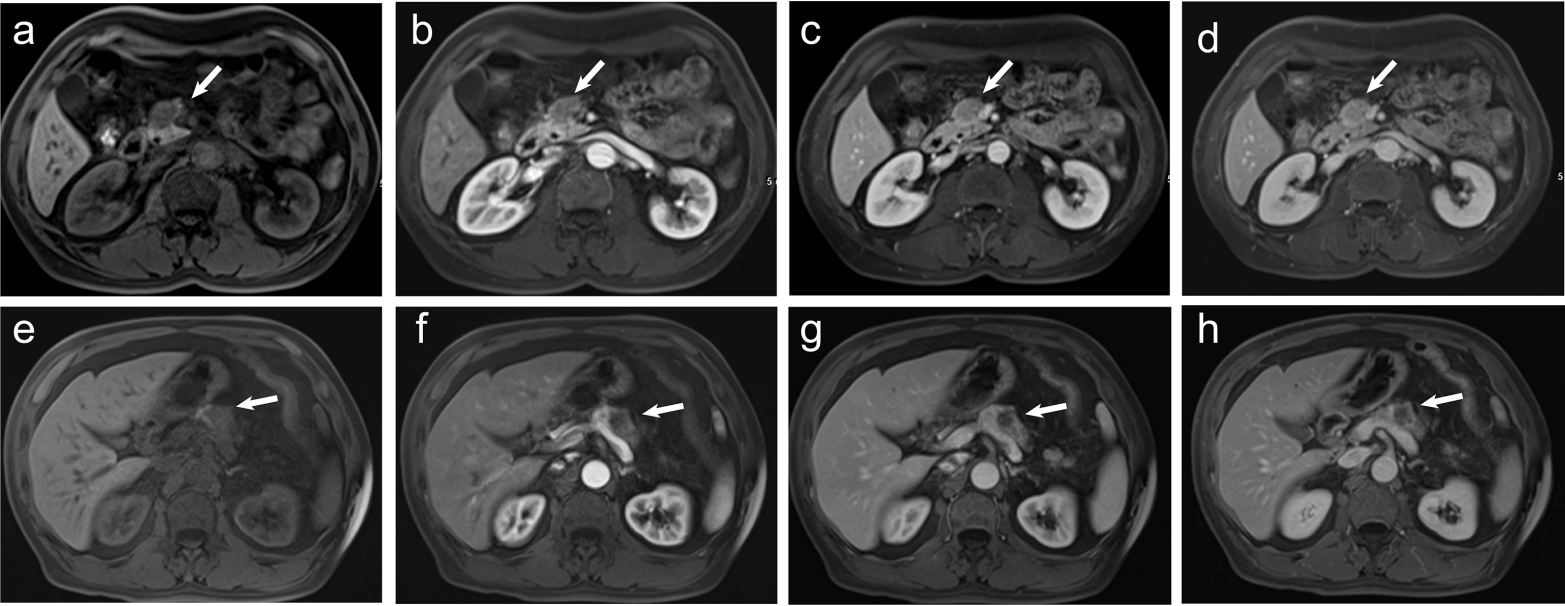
(A–D) A 60-year-old man pathologically diagnosed with G2 non-hypervascular PNETs. (A) T1-weighted imaging shows a well-defined hypointense mass in the head of the pancreas (white arrow). (B) The mass in the arterial phase shows hypointensity (white arrow). (C, D) The mass in both portal (C) and delayed phases (D) shows relative isointensity (white arrow). (E–H) A 60-year-old man pathologically diagnosed with PDACs. (E) T1-weighted imaging shows an ill-defined hypointense mass in the body of the pancreas (white arrow). (F) The mass in the arterial phase shows hypointensity (white arrow). (G) The mass in the portal phase shows obvious hypointensity with mild pancreatic duct dilation (white arrow). (H) The mass in the delayed phase shows obvious hypointensity (white arrow).
